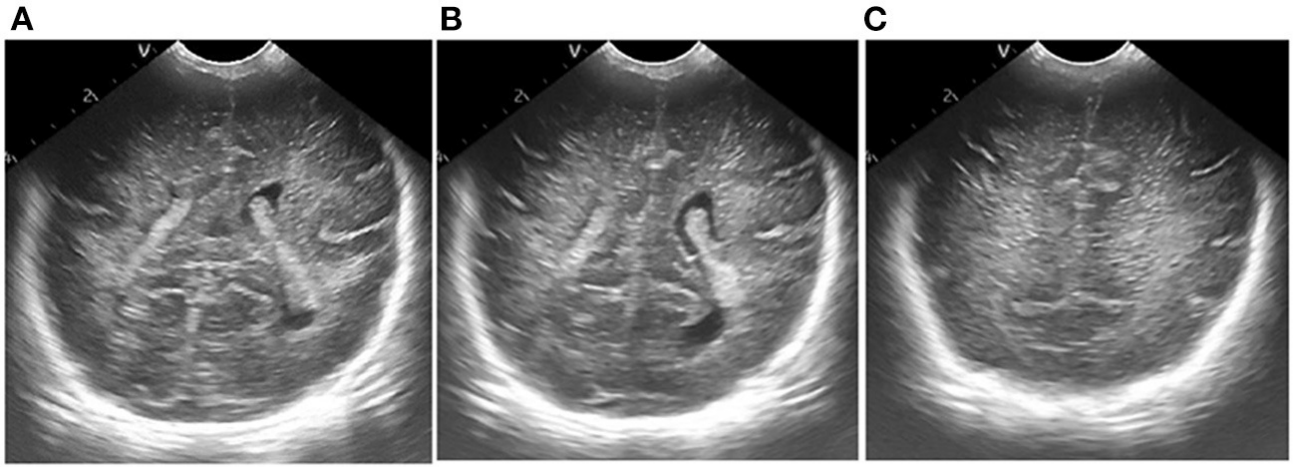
Results of ultrasonic image clipping. (A) Plane 1, (B) plane 2, (C) plane 3.
